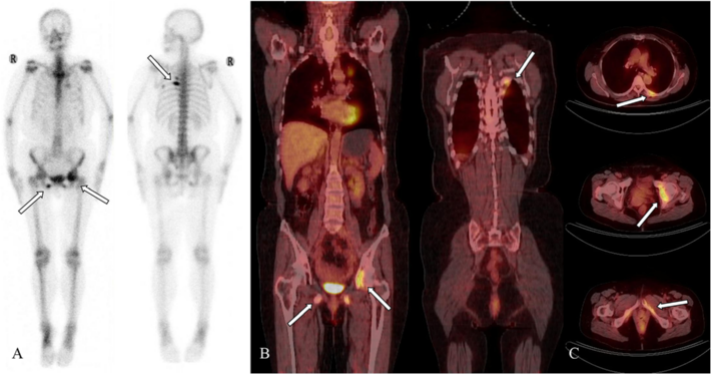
A 61-year-old woman with left superior lung cancer. Bone scanning (BS) indicated focally increased uptake in the left fifth and sixth posterior rib, the left acetabulum, and the bilateral inferior ramus of the pubis (A). PET-CT revealed that the bone in the left fifth and sixth posterior ribs, the left acetabulum, and the bilateral inferior ramus of the pubis was destroyed. ^18^FDG uptake in these areas was significantly increased. The maximum standardized uptake value was 5.25 (B and C). The same number of metastatic lesions were detected using PET-CT and BS.
